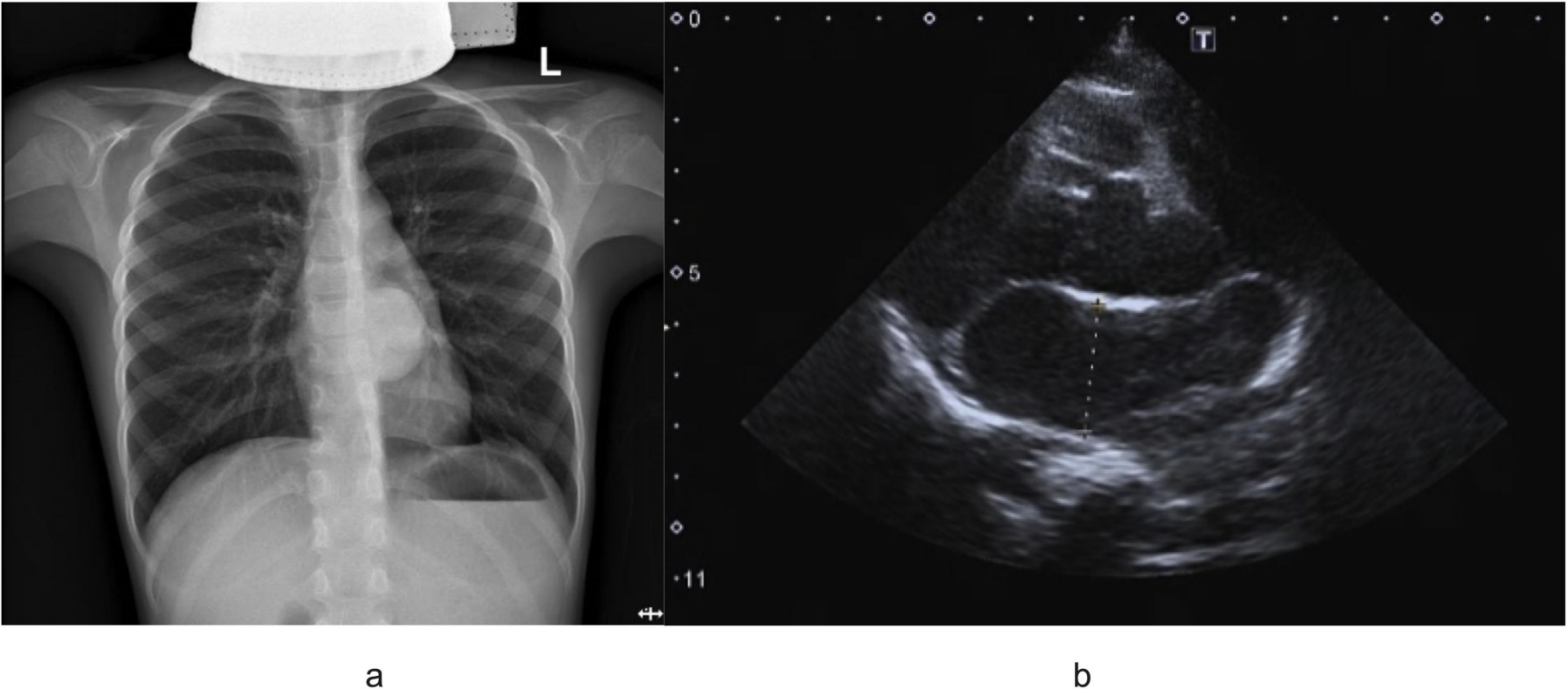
(a) Outcomes of preoperative chest radiograph demonstrated a high-density mediastinal shadow; (b) preoperative ultrasound examination indicated left posterior mediastinal mass lesion.
